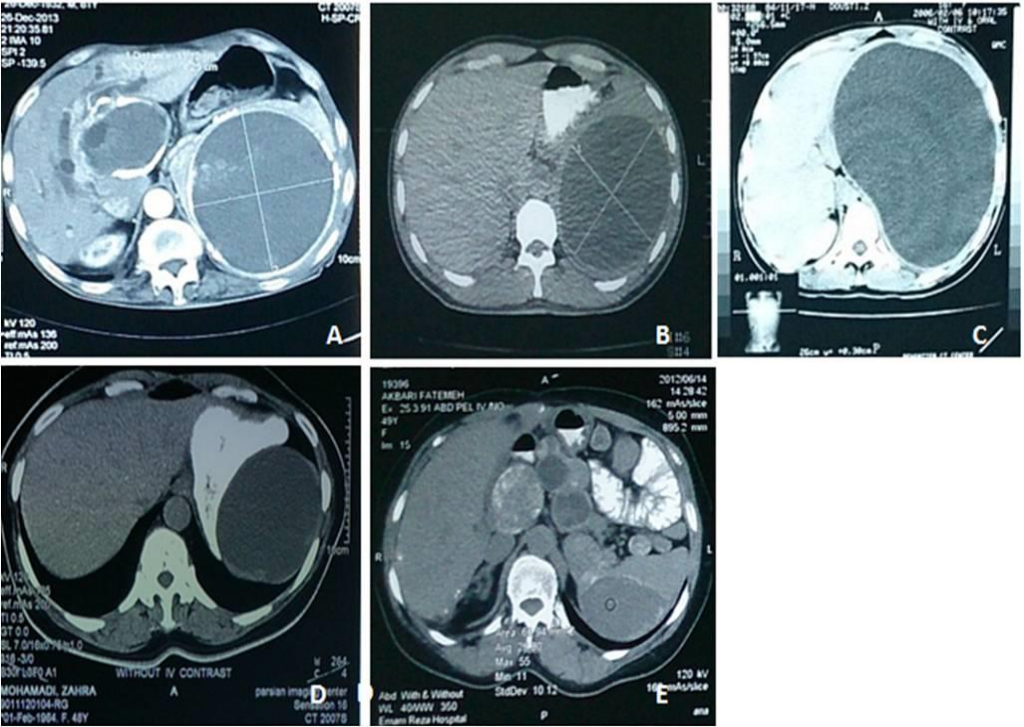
remarkable CT-scan findings in our trial;
